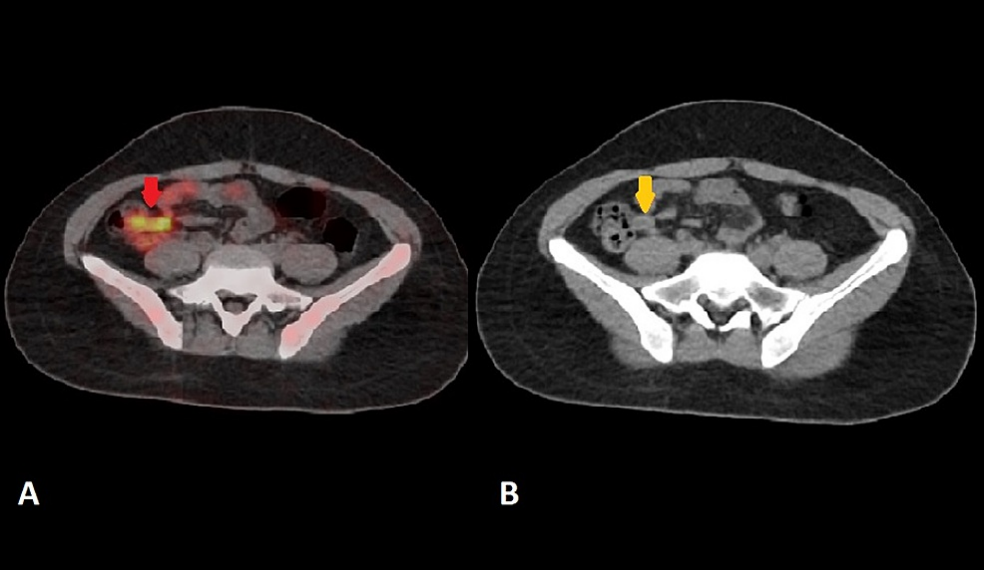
18F-FDG PET/CT axial images: (A) fused image (B) CT image (A) Fused image shows 18F-FDG avidity projecting over the appendix (red arrow) (B) CT image shows a fluid-filled dilated appendix with mural thickening (yellow arrow). No evidence of periappendicular fat stranding 18F-FDG PET/CT: Fluorine-18-Fluorodeoxyglucose Positron Emission Tomography/Computed Tomography
